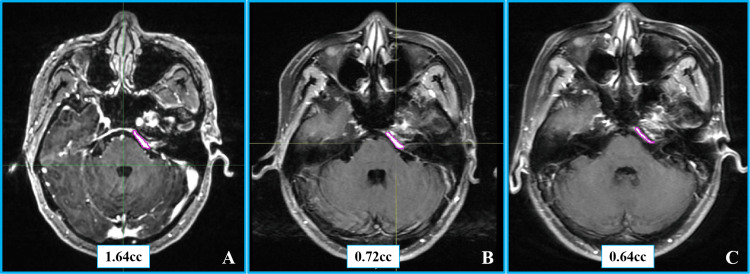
Contrast-enhanced MRI images of Case 1. (A) Axial contrast-enhanced T1-weighted image with contouring of the tumor volume before radiosurgery. (B) The same sequence with tumor volume contouring one year post-ZAP-X radiosurgery. (C) Axial contrast-enhanced T1-weighted imaging with tumor volume contouring four years post-ZAP-X radiosurgery.
